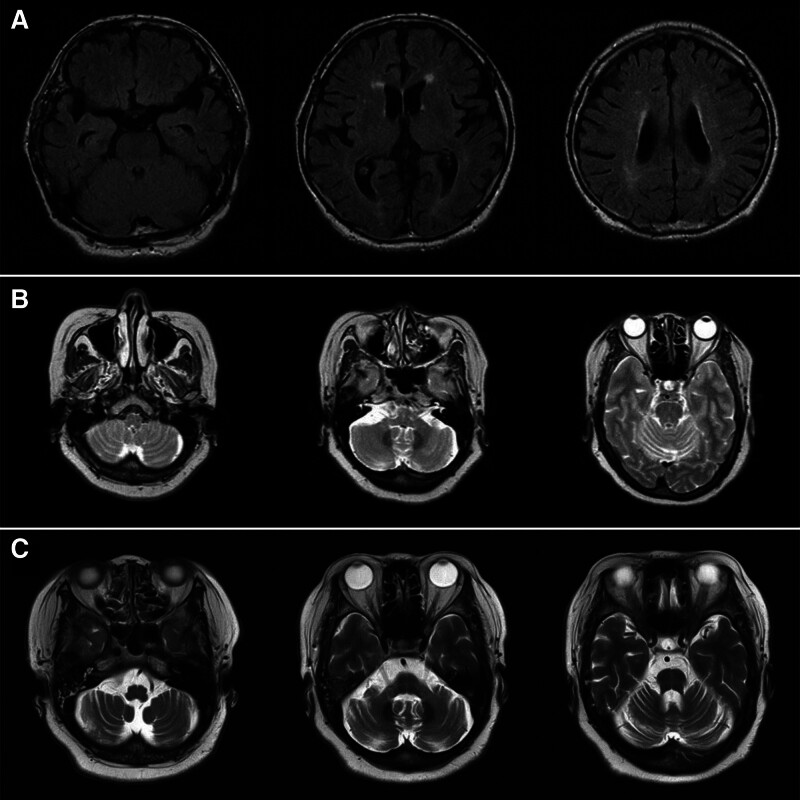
Brain MRI findings of the patients. (A) Case 1’s brain MRI T2 FLAIR axial images showing mild periventricular small vessel disease (age 77). (B) Case 2’s brain MRI T2 axial images showing mild diffuse cerebellar atrophy (age 52). (C) Case 2’s follow-up images showing progressed cerebellar atrophy and pontine atrophy (age 55). FLAIR = fluid-attenuated inversion recovery, MRI = magnetic resonance imaging.
